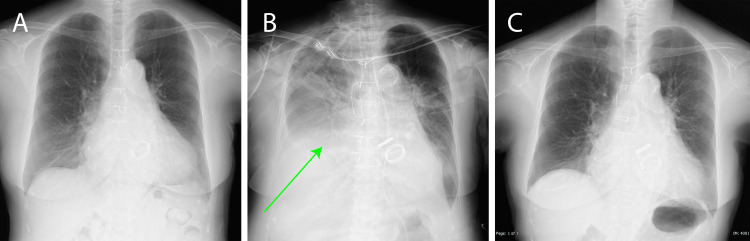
Chest X-ray imaging findings (A) Preoperative. (B) Post operative. Right diaphragm elevation (green arrow) and post-tracheostomy tube identified. (C) One year after the operation. Elevation of the right diaphragm had improved.
